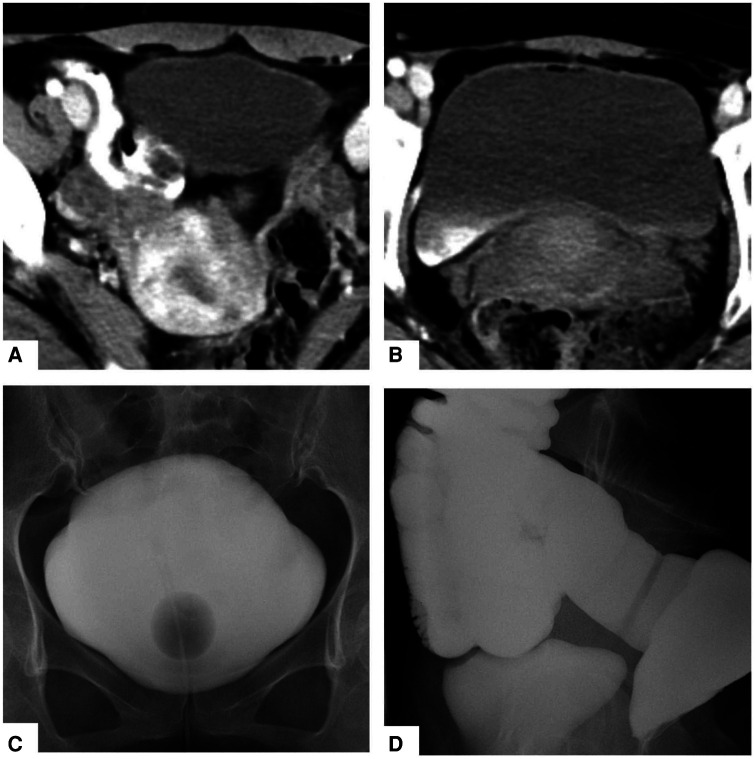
Abdominal CT revealed that a small amount of contrast agent had drained into the bladder from the small intestine, and there was a small amount of air in the bladder (A,B). Cystography showed that no contrast medium entered the small intestine from the bladder (C), but enterography clearly showed that a large amount of contrast medium had entered the bladder from the small intestine (D).
